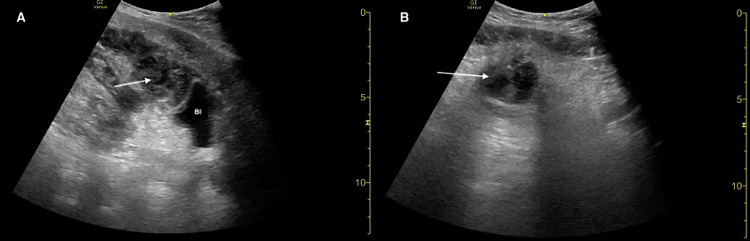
Point-of-care ultrasound demonstrating a Crohn’s disease-related intra-abdominal abscess (arrows) in sagittal (A) and transverse (B) planes. Bl: bladder.
